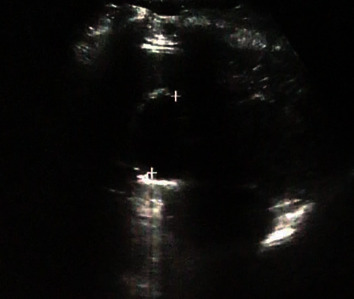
Ultrasound of retroperitoneal MLS patient. Retroperitoneal MLS, indicated by while “+” symbols.
